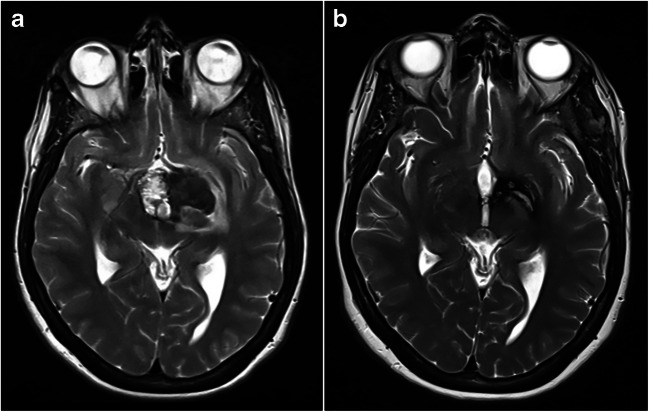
Axial T2WI head-MRI scan. a Pre-operative head-MRI scan. CCM located between the hypothalamus, the third ventricle, and the perimesencephalic cisterns with recent bleeding causing mass effect and third ventricle displacement of 7 mm. b Post-operative head MRI scan. Post-surgical malacic area at the level of the left lenticular nucleus; in the interpeduncular cistern nodule with an axial diameter of 10 × 4 mm compatible with residual cavernoma
